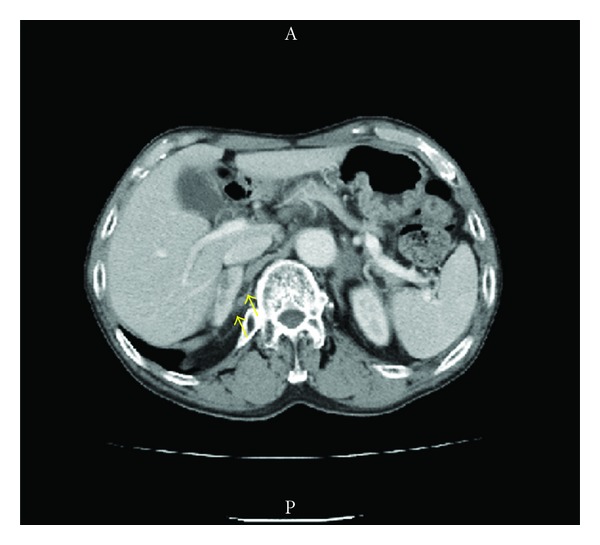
Computed tomography at 6 months after the left adrenalectomy shows right adrenal tumor (5.0 × 1.8 cm).
